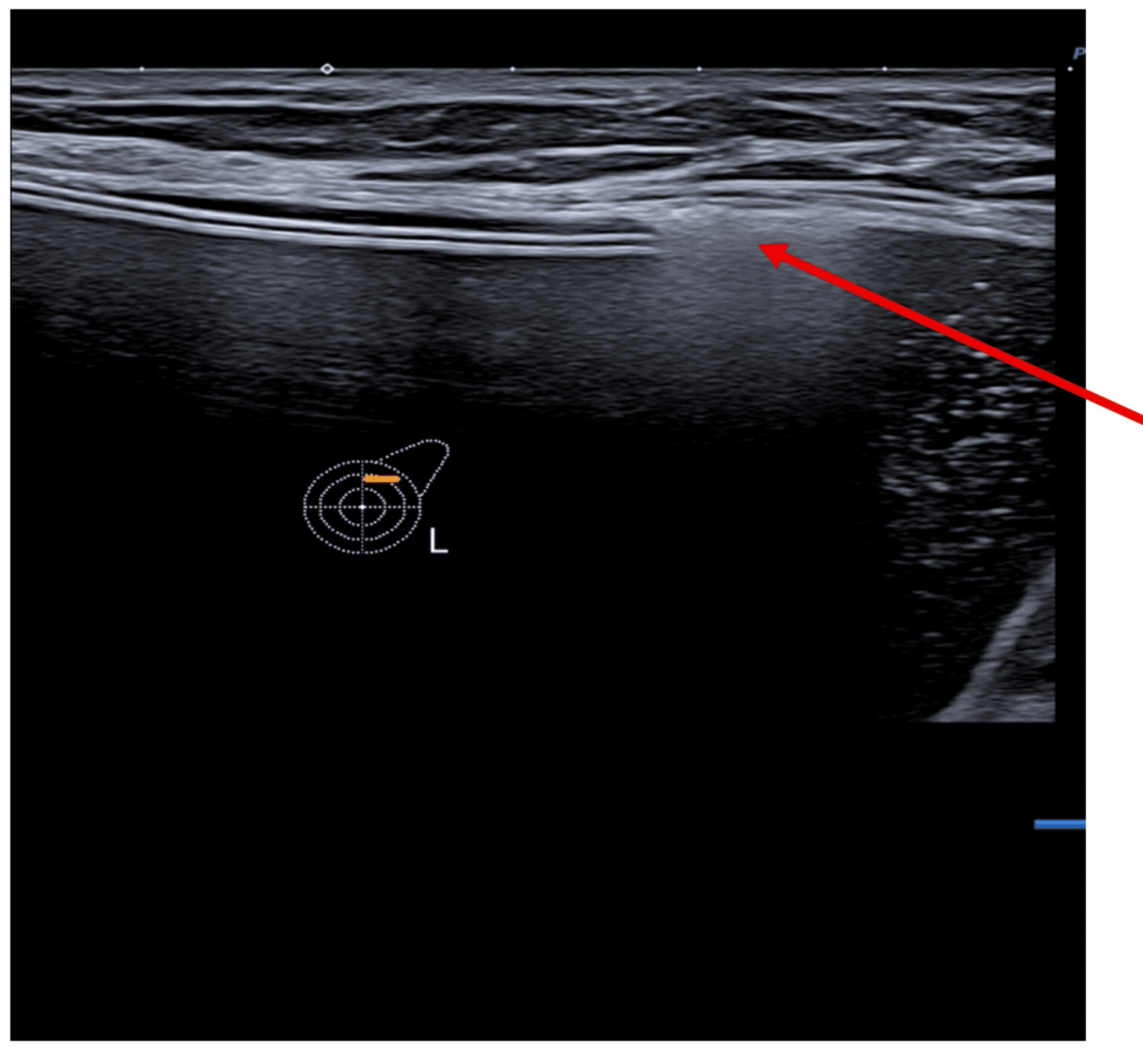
Ultrasound scan of the left breast revealed a single lumen implant with peri-implant echogenicity representing the intracapsular rupture (arrow).
